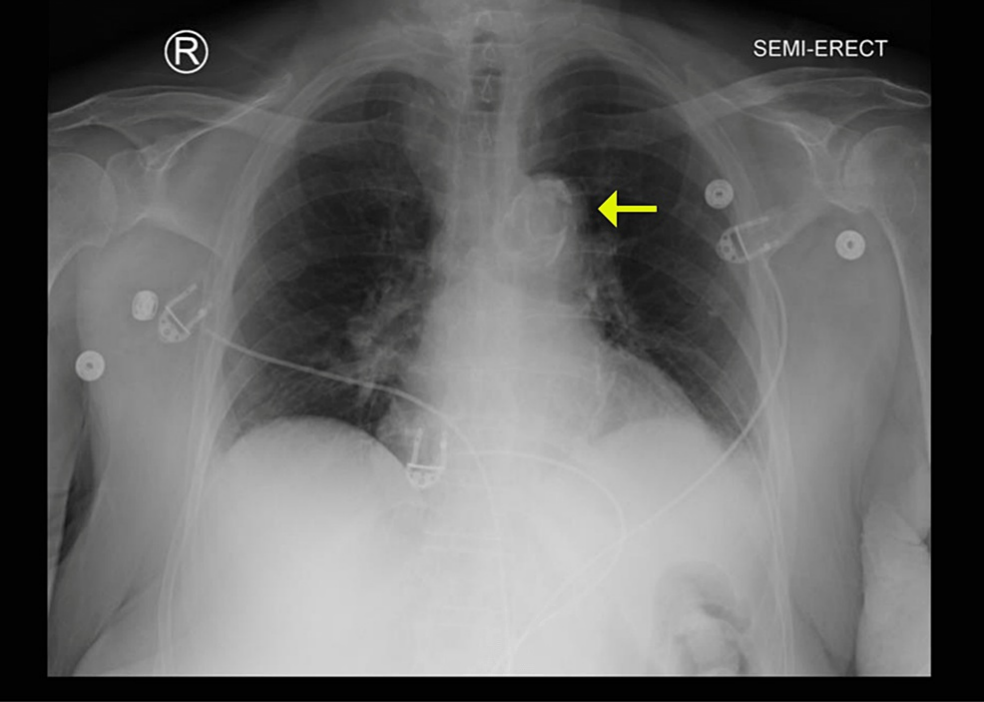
Chest Radiograph Chest X-ray showing unidentified object in the upper mediastinum (arrow).
